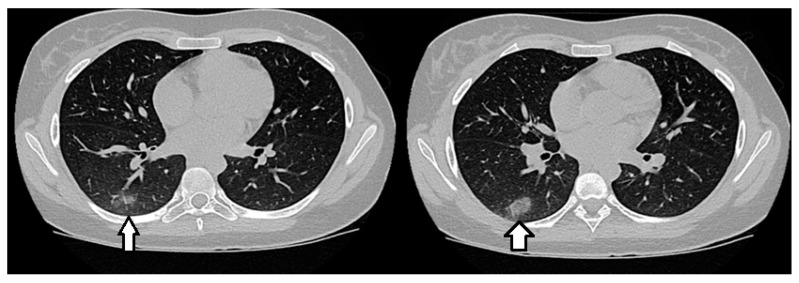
CT scans of the chest during the first relapse, showing ground opacities (white arrows) in the right lower lobe.
